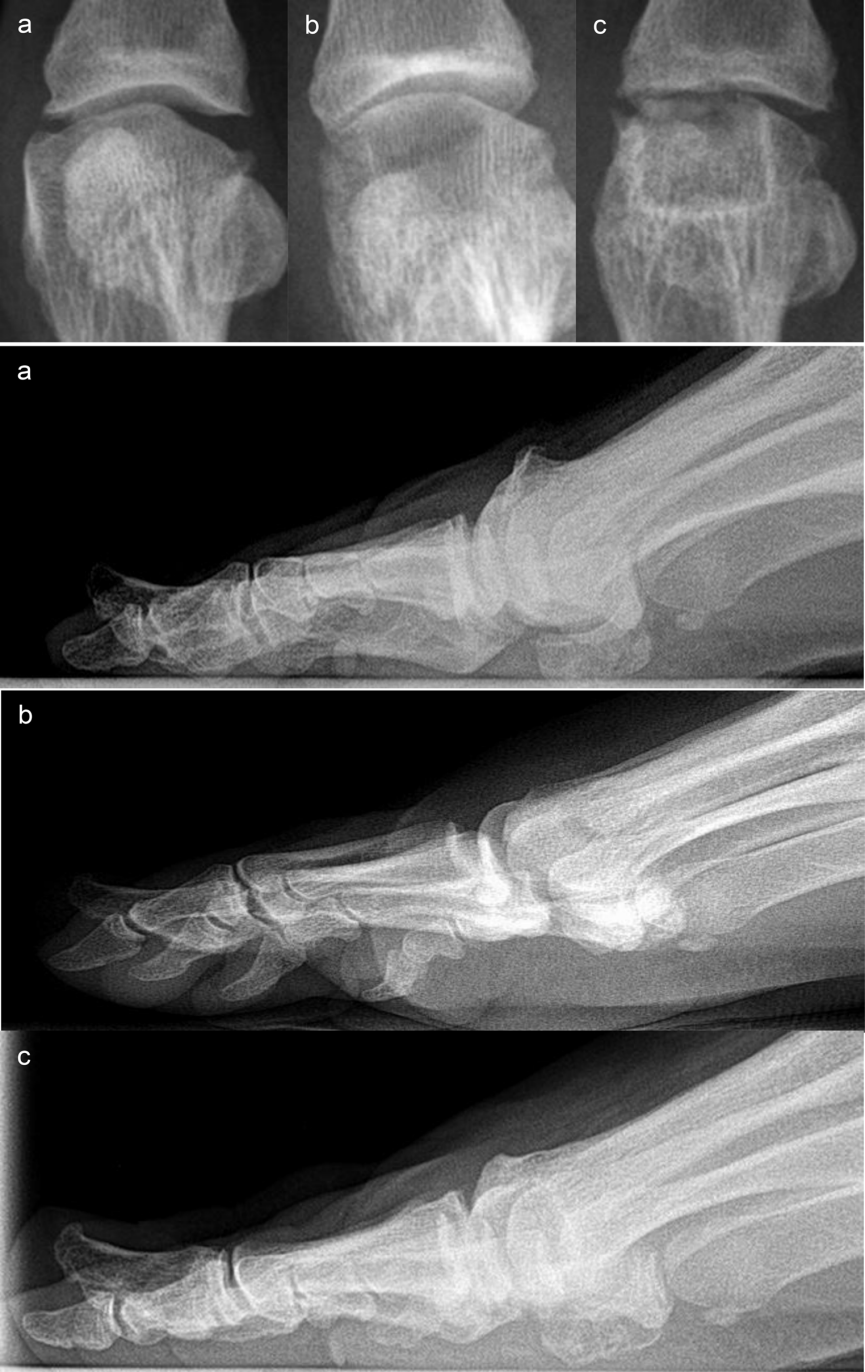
Case with pronounced bright sclerotic margin around the implant a: Preoperative condition. b: Postoperative control radiograph: Regular position of the bone canal c: Follow-up at 21 months postoperatively, severe bright sclerotic margin and severe erosive changes on the bone surrounding the implant and the articular surface of the proximal Os metatarsale I
